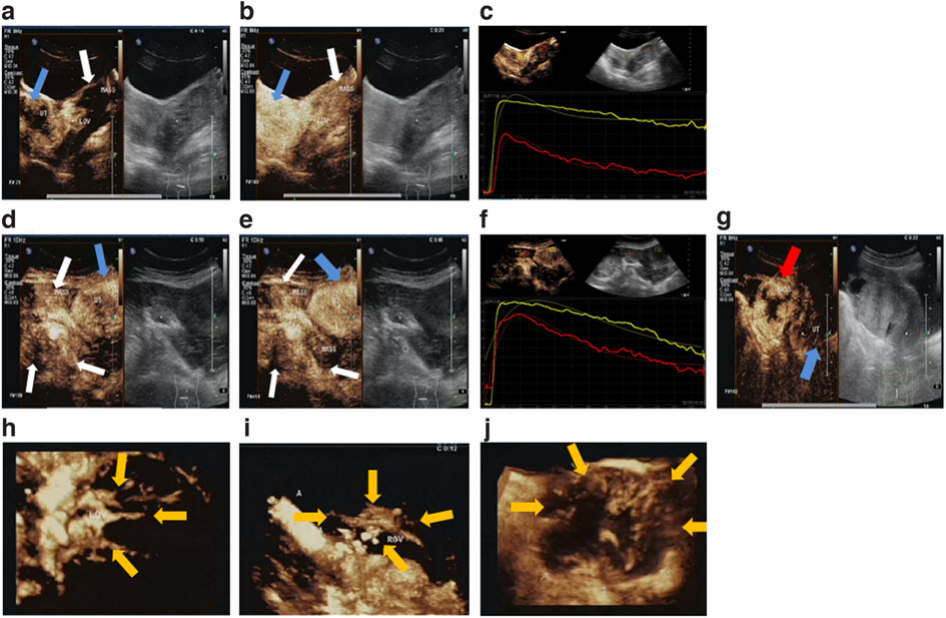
CEUS features of OSSPBT. (a–c) 2D-CEUS: the mass (white arrow) around the left ovary began to strengthen later than the uterine wall (blue arrow) (a), and the PI of the mass (white arrow) was lower than that of the uterine wall (blue arrow) (b). The contrast TIC curve of the lesion and myometrium (c) red is the lesion, yellow is the myometrium. (d–f) 2D-CEUS: the mass (white arrows) around the right ovary and the uterine wall (blue arrows) were in a synchronous low enhancement (d), and the regression rate was faster than that of the uterine wall (blue arrows) (e). The contrast TIC curve of the lesion and myometrium (f) red is the lesion, yellow is the myometrium. (g) The 2D-CEUS of MOSC (red arrow) showed that the centripetal enhancement was earlier than that of uterine wall (blue arrow). (h, i) 3D-CEUS: the trophoblastic vessels of the tumor originate from the left ovary and are radial branches (yellow arrows) (h). The tumor is supplied by large branching blood vessels around the right ovary (yellow arrows) (i). (j) The 3D-CEUS of MOSC showed irregular branches of nutrient vessels (yellow arrows) from the periphery to the center. 2D, two-dimensional; 3D, three-dimensional; CEUS, contrast-enhanced ultrasound; PI, peak intensity; TIC, time–intensity curve.
